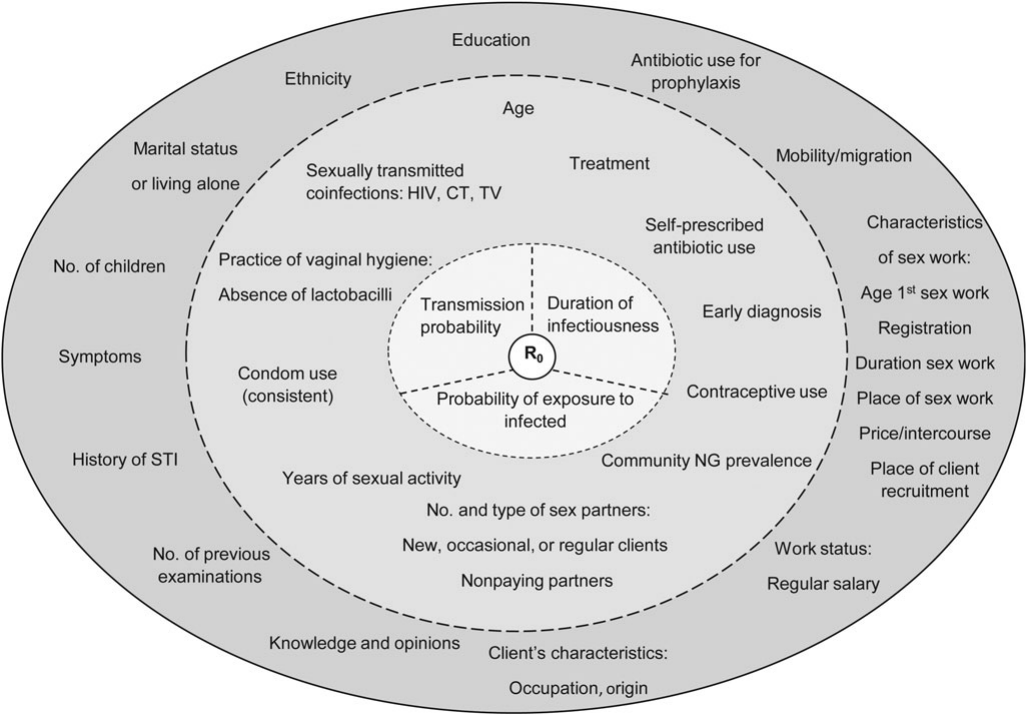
Proposed framework of gonorrhea risk from studies of female sex worker populations. R_0_, the reproductive number, is at the center of the figure. At the first level, directly influencing R_0_, are its 3 components: probability of exposure to infected, transmission probability, and the duration of infectiousness. At a second level, we positioned all the proximate determinants that influence each of the components of R_0_. At a third level, there are the distal/underlying determinants. When different types of measures or proxies of the same determinant were found in the literature, we indicated the determinant and proceeded to list the different measures found (eg, characteristics of sex work: age at first sex work, duration of sex work, registration, place of sex work, place of client recruitment, price per intercourse). Abbreviations: CT, *Chlamydia trachomatis*; HIV, human immunodeficiency virus; NG, *Neisseria gonorrhoeae*; No, number; R_0_, reproductive number; STI, sexually transmitted infection; TV, *Trichomonas vaginalis*.
